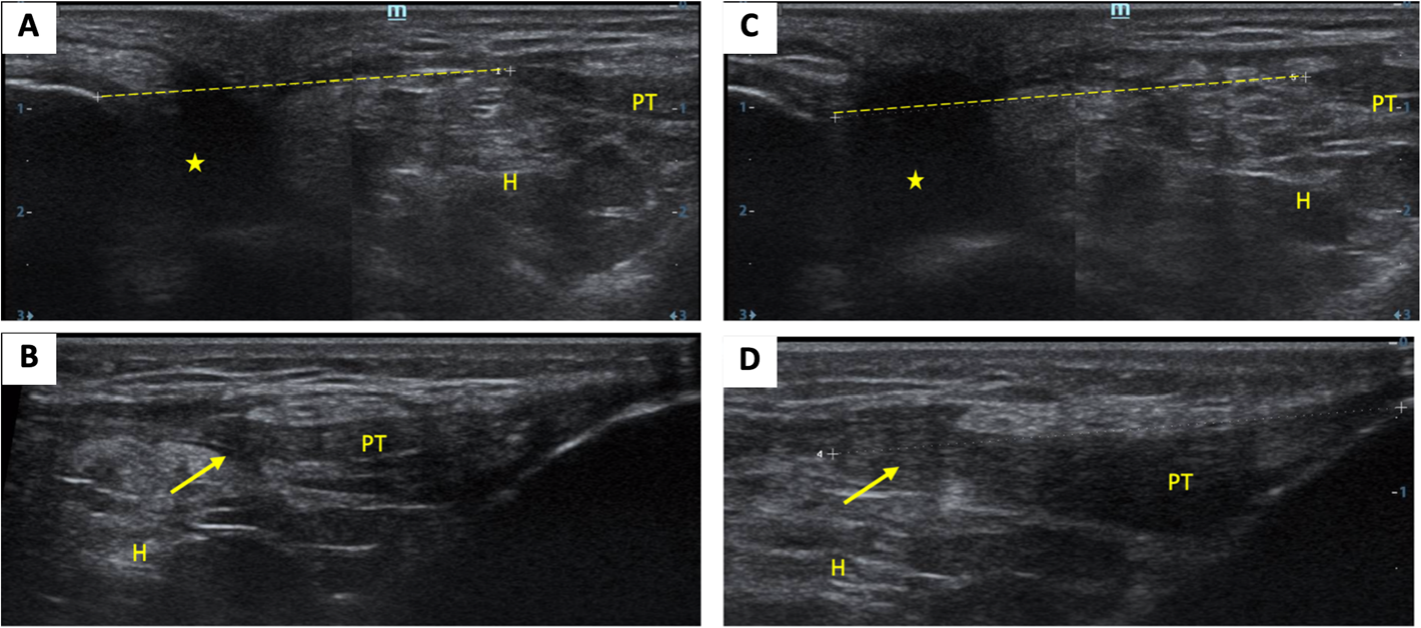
Ultrasound of the knees performed using a high frequency linear transducer. a and b: right knee. c and d: left knee. Median sagittal sections of the patellar tendons (PT) showing the stump of the patellar tendon (arrow) and the diastasis between the apex of the patella and the stump (discontinuous line), associated with an effusion of the suprapatellar bursa (yellow star) as well as an infiltration of Hoffa’s fat pad (H)
